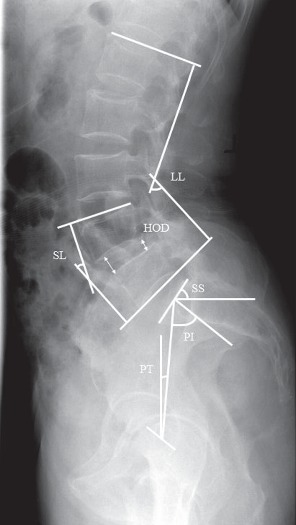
Measurement of spinopelvic sagittal balance (lumbar lordosis, segmental lordosis, sacral slope, height of the intervertebral disc, pelvic incidence, and pelvic tilt) on a lateral radiograph.
